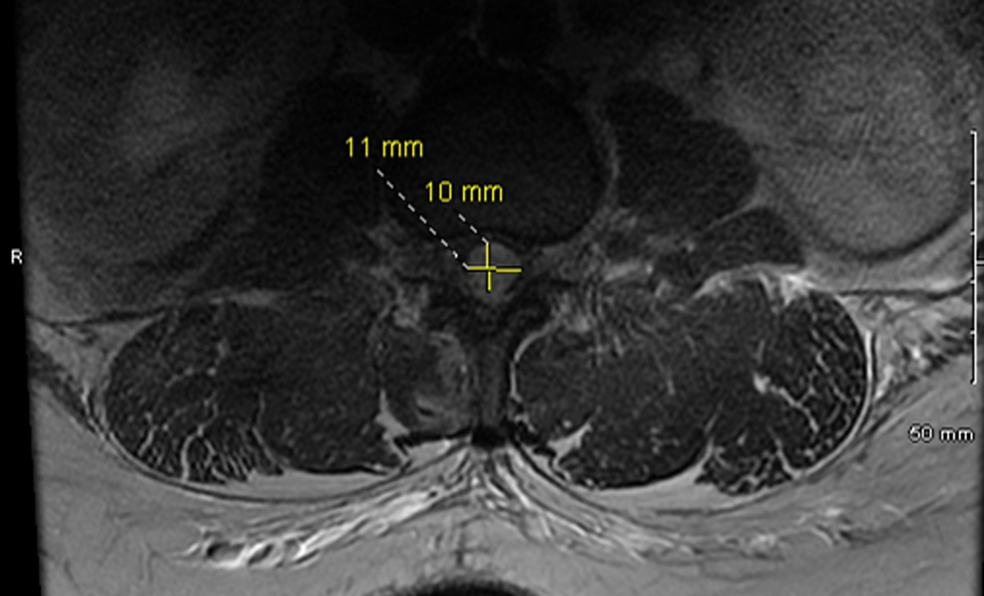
T2 axial MRI of the lumbar spine without contrast showing the transverse view of the hematoma measuring 1.1 cm x 1 cm MRI, Magnetic resonance imaging.
